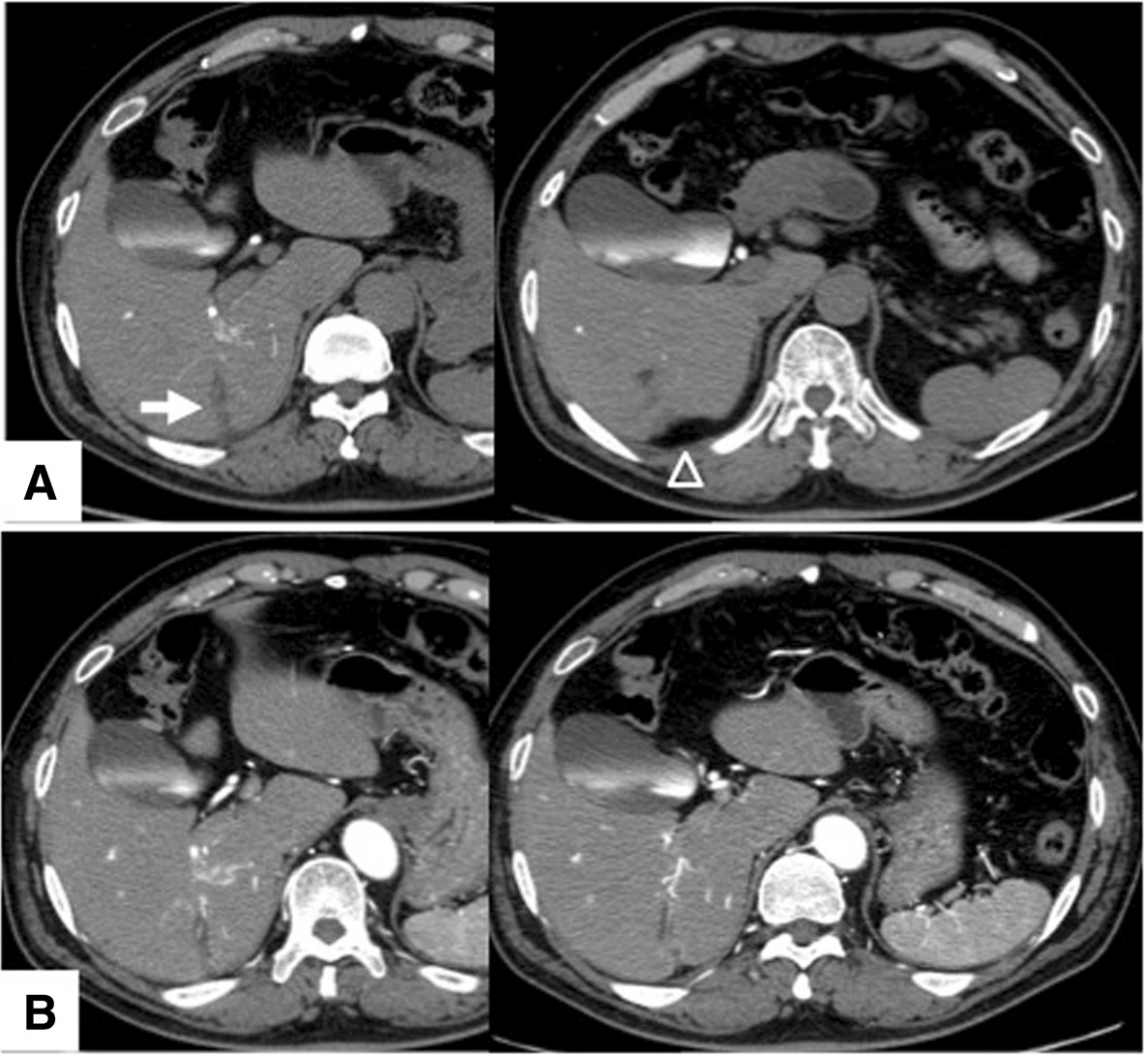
Computed tomography findings. a Dynamic computed tomography with drip infusion cholangiography revealed focal dilatation of the biliary ducts (*arrow*) and atrophy (*arrowheads*) in the posterior segments of the liver. b No tumor was detected via enhanced computed tomography
